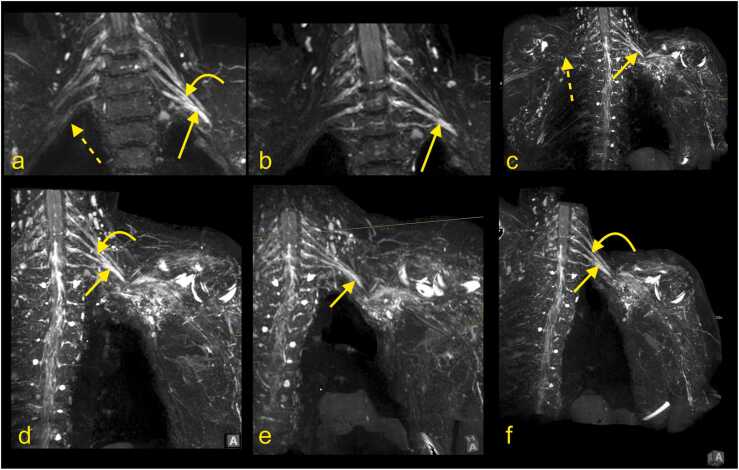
Continuation of the patient from the previous figure. MRI neurography (a-e) with 3D- Maximum Intensity Projection (MIP) reconstructions (d-e) showed the discreet asymmetry of the BP; the left one is more prominent (straight arrows show the lower trunk, the curved arrows show the middle trunk, the straight dashed arrows show the normal BP). The normal signal of the BP is not visible on the level of the radiation fibrosis. However, no discontinuity was demonstrated.
